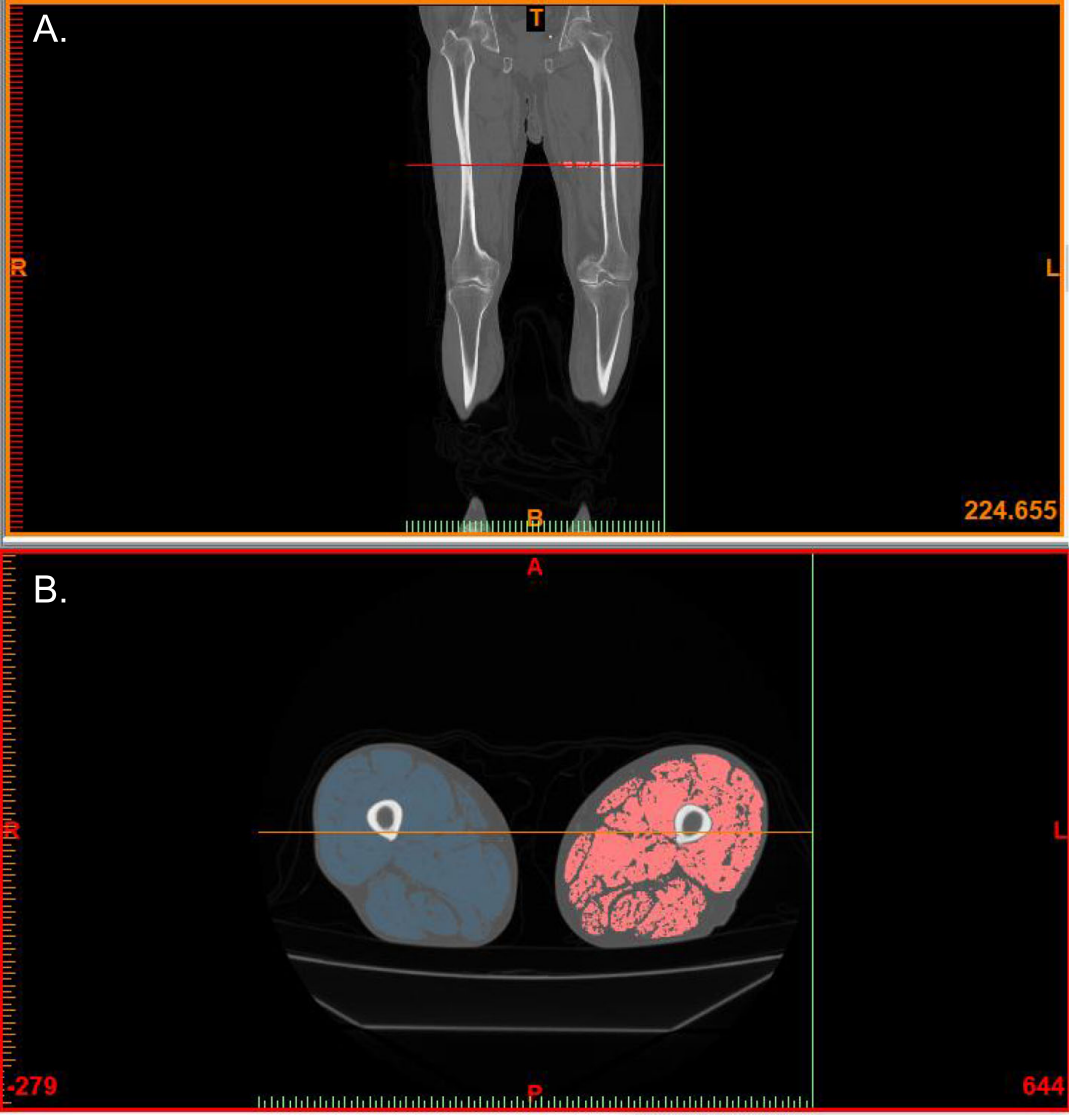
Estimation of muscle volume using CT-image analysis. a CT image of both legs of a patient. Red line indicates the location of a digital slice taken approximately 200 mm above the knee joint. b CT images of a cross section of bilateral muscles were semi-automatically analyzed in a 10-mm region of interest (ROI) using the MIMICS® software
